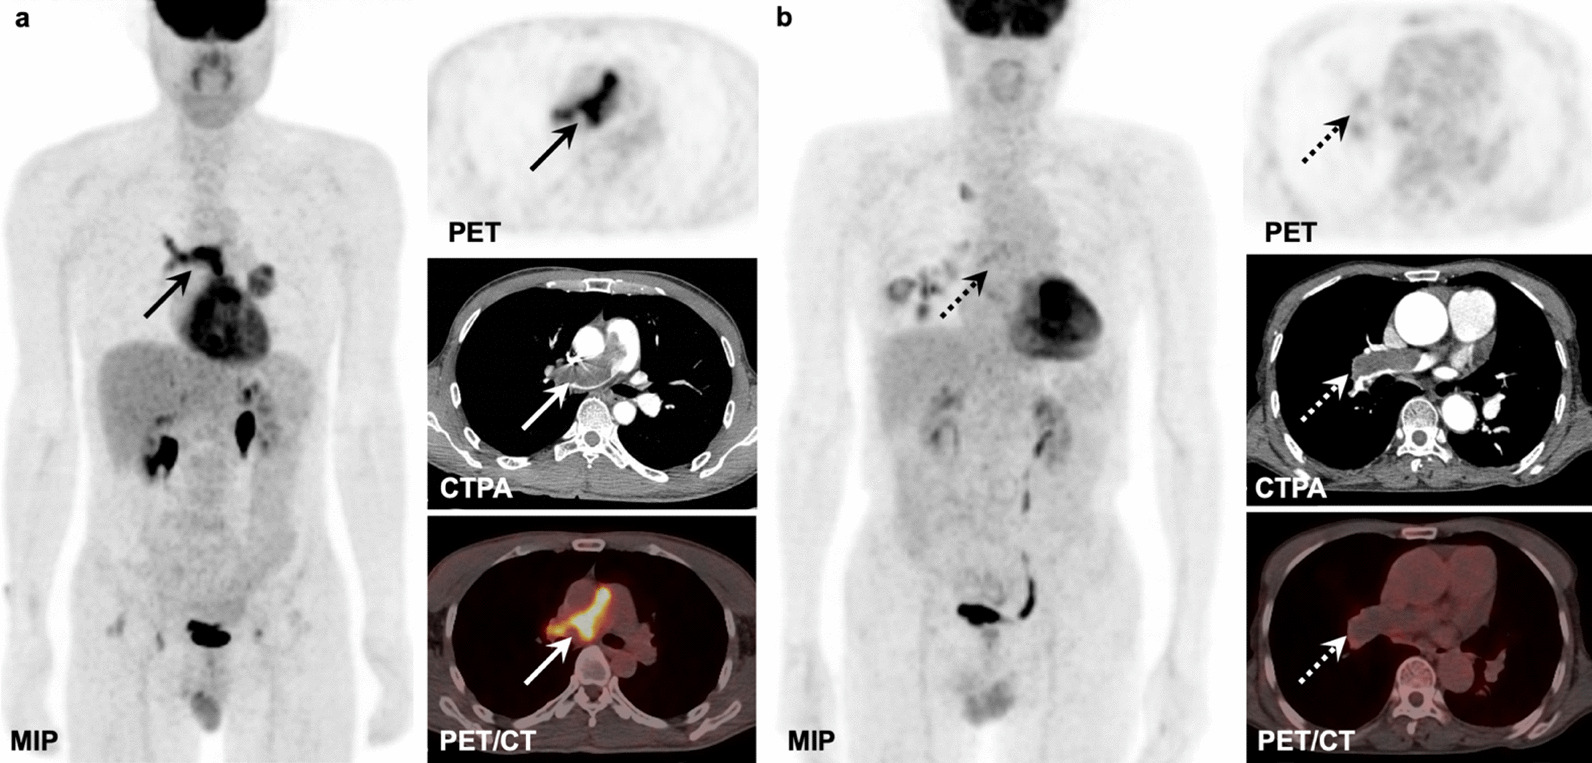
a PAS in a 52-year-old man involving PT and RPA (solid arrows) with intense FDG uptake (SUVmax: 10.7) on the MIP, axial PET, and fused PET/CT images, and filling defect on the CTPA image. b PTE in a 64-year-old man involving RPA (dotted arrows) with mild FDG uptake (SUVmax: 1.1) on the MIP, axial PET, and fused PET/CT images, and filling defect on the CTPA image
